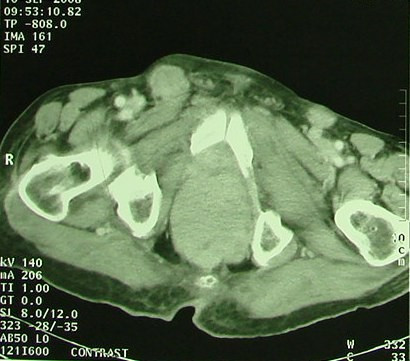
Pelvic computed tomography scan performed before chemotherapy treatment shows the Bulky anorectal process (see arrows).
